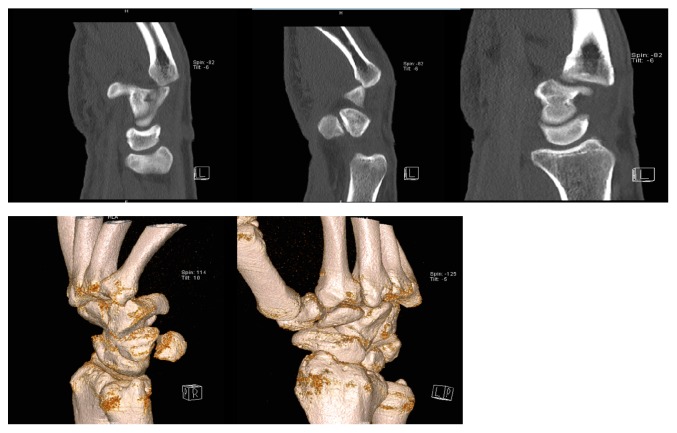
Complementary CT scan showing no other lesions than complete dislocation of the fourth and fifth CMC joints and a partial dislocation of the third CMC joint.
